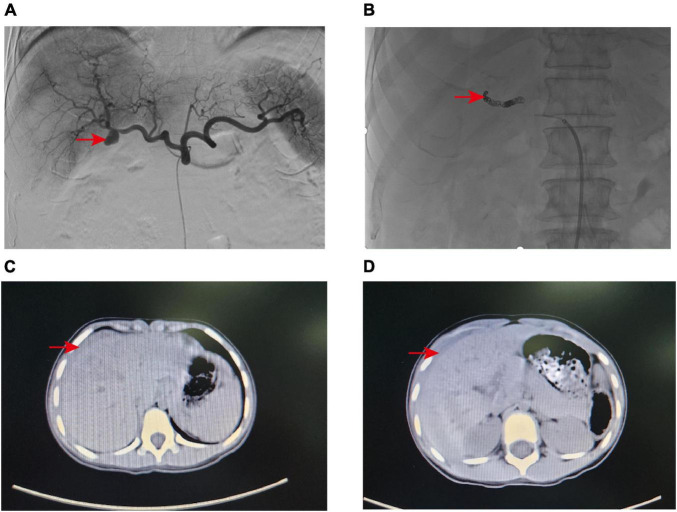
(A) Angiography revealed a pseudoaneurysm at the distal end of the right hepatic artery trunk. (B) The pseudoaneurysm was successfully embolized with Microcoils. (C,D) CT scan indicated that the pediatric patient had liver contusion with surrounding hematoma formation.
